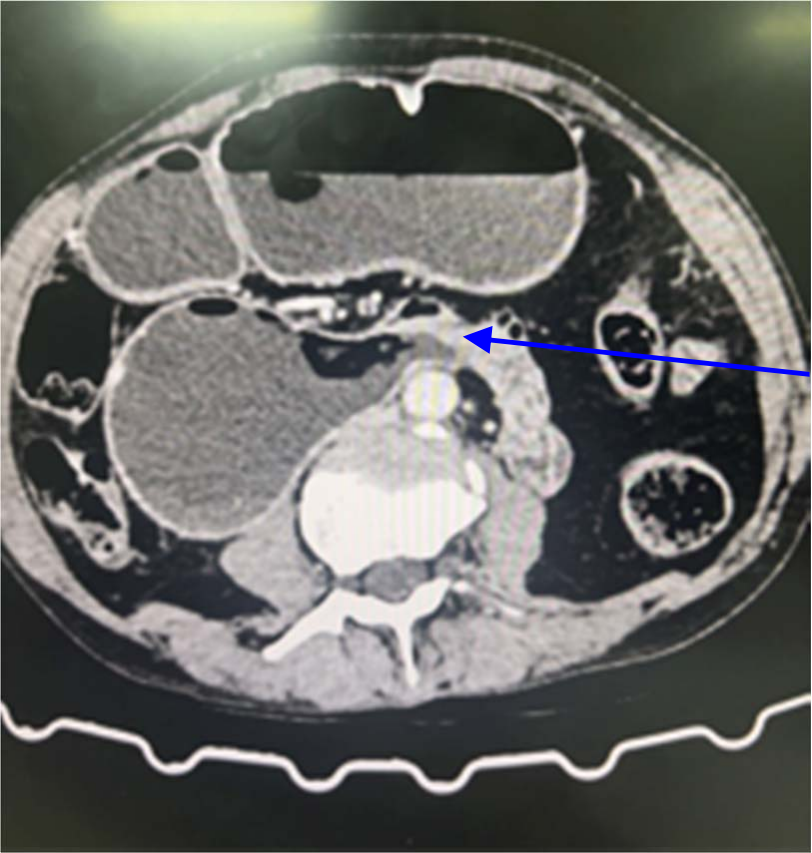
Computed tomography scan axial section showing homogenous, circumferential thickening at duodenojejunal flexure spanning 2.2 cm and thickness of 11 mm.
